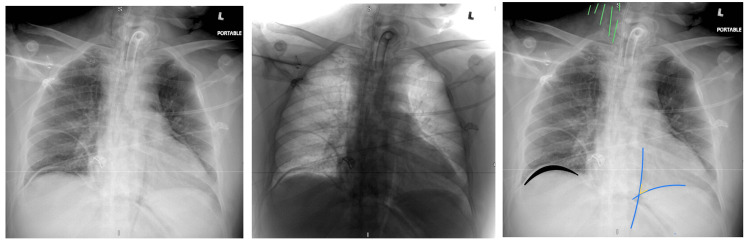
Portable chest X-ray done to confirm the position of the tracheostomy tube and rule out complications Tracheostomy and nasogastric tubes are in place. The lower right neck soft tissue appears heterogeneous with areas of ill-defined linear and nonlinear lucencies: subcutaneous emphysema (represented by the green marks). Air under the diaphragm on the right side can be seen: pneumoperitoneum (represented by a black marking). Multitudes of thin streaks can be seen in the mediastinum. Air can be seen along the left heart border (represented by red markings). Lucent band of gas that extends along the descending aorta and intersects the band that extends along the medial left hemidiaphragm, together forming a “V”: Naclerio’s V sign (represented by the yellow marking). Although this finding was originally described in association with esophageal rupture, it is not specific to this condition.
